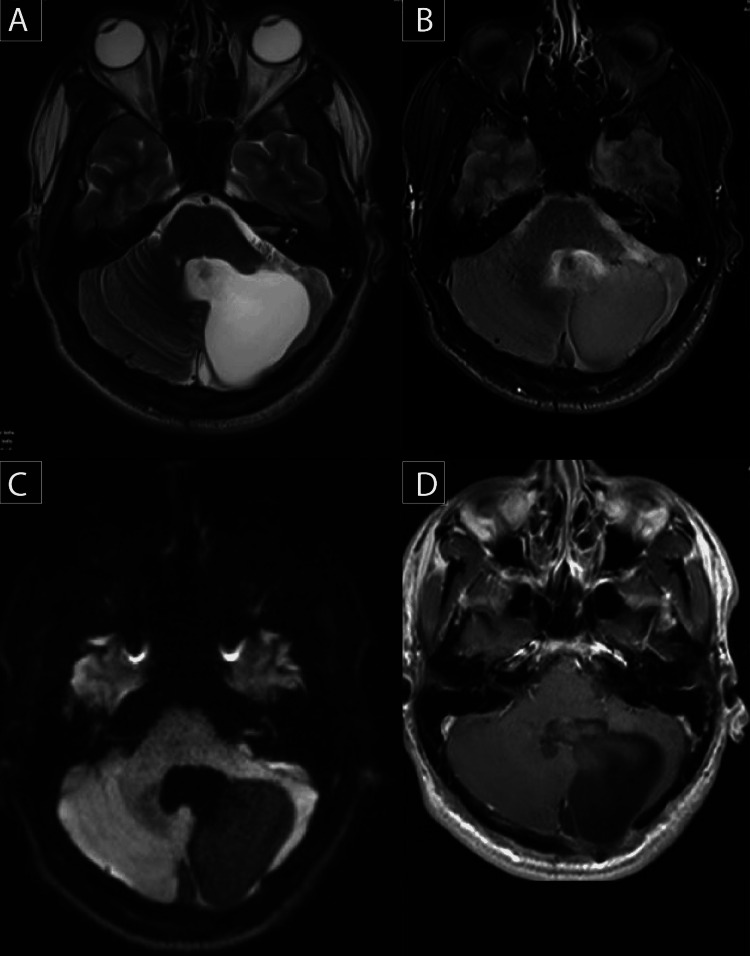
(A) T2-weighted image. (B) FLAIR. (C) Diffusion-weighted image. (D) Axial T1 post-contrast. FLAIR: Fluid-attenuated inversion recovery.
